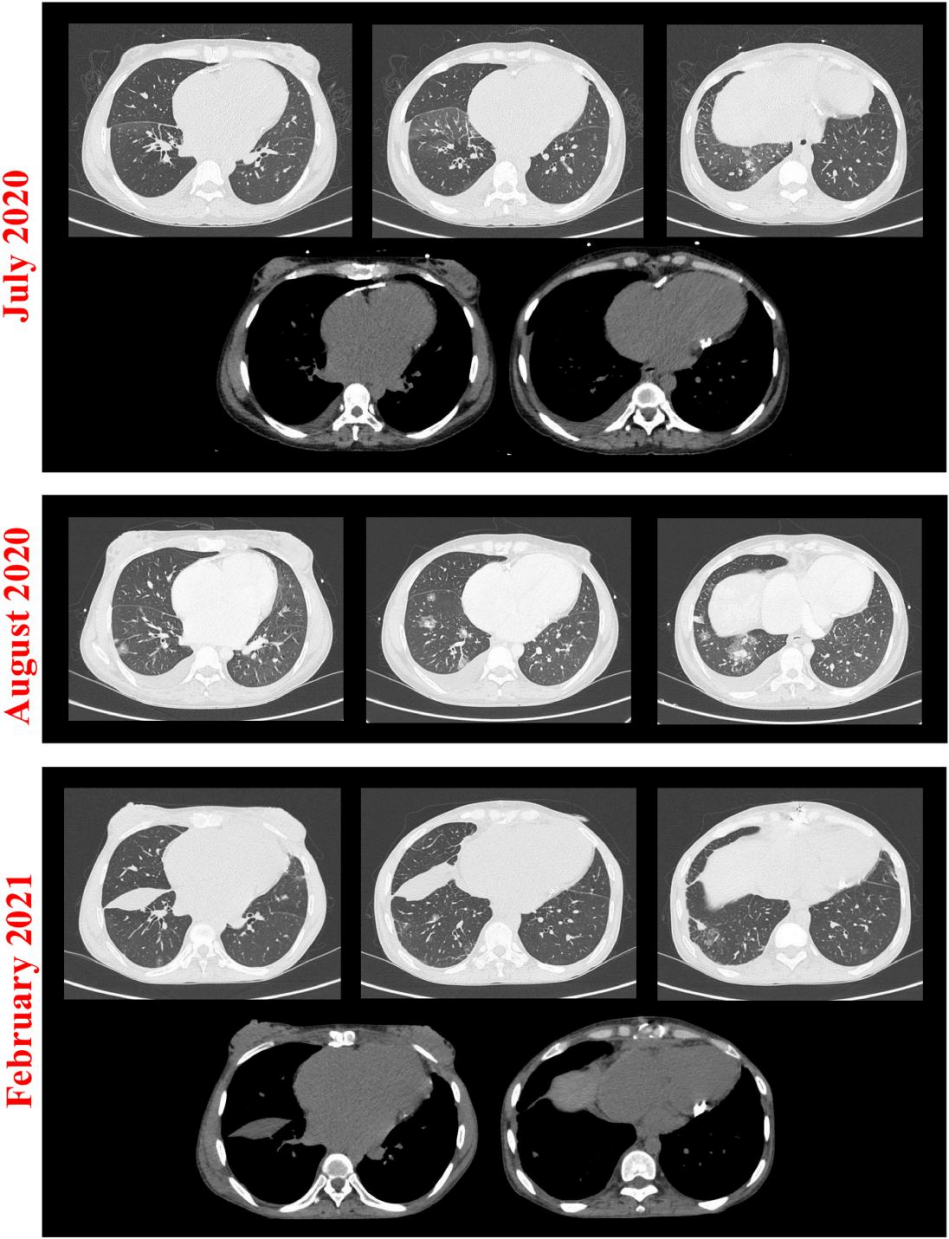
Pulmonary manifestations. Pulmonary window thoracic computed tomographies (top of image subsets) demonstrating multiple bilateral nodules migrating over time (areas of alveolar haemorrhage) and right-sided infrascissural pleural effusion (February 2021) consistent with pleuritis; mediastinal window thoracic computed tomographies (bottom of image subsets) showing pericardial calcifications pre- (July 2020) and post-pericardiectomy (February 2021).
